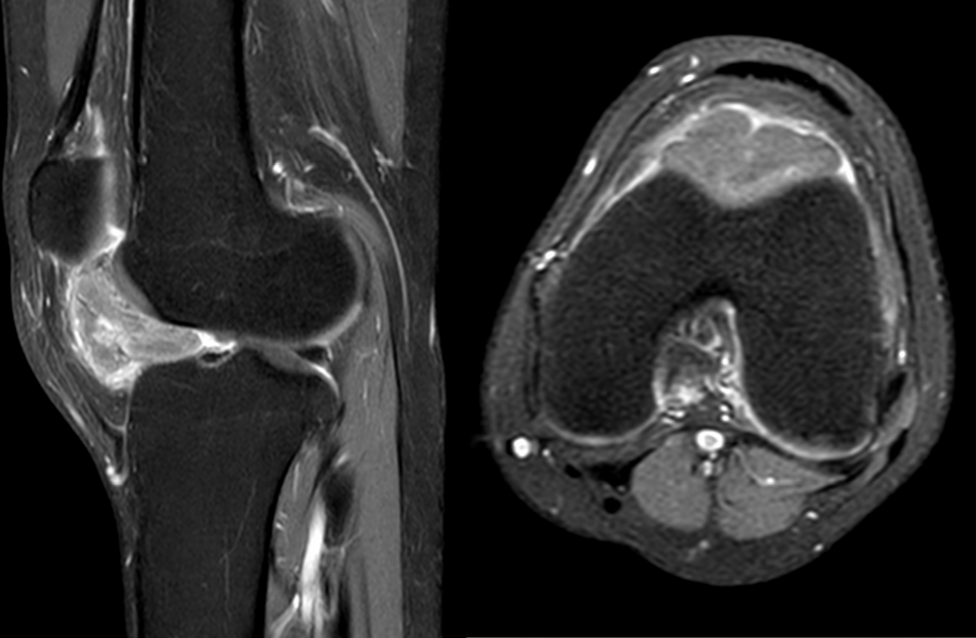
Sagittal (left) and axial (right) post IV with paramagnetic contrast medium, T1-weighted, with fat suppression, magnetic resonance images showed a lobulated intra-articular mass within Hoffa’s fat pad. The mass exhibited mild heterogeneous contrast uptake
